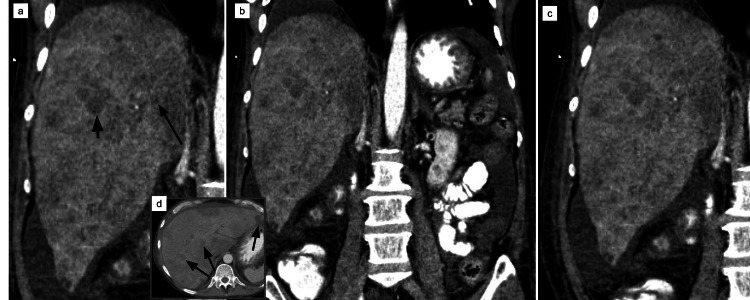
computed tomography (CT ) abdomen and pelvis with contrast (a,b,c - coronal view, d- axial view ) showing diffused innumerable hypodense nodules involving both lobes of the liver in various plains. The thick arrow denotes the largest and the thin arrows smaller nodules.
